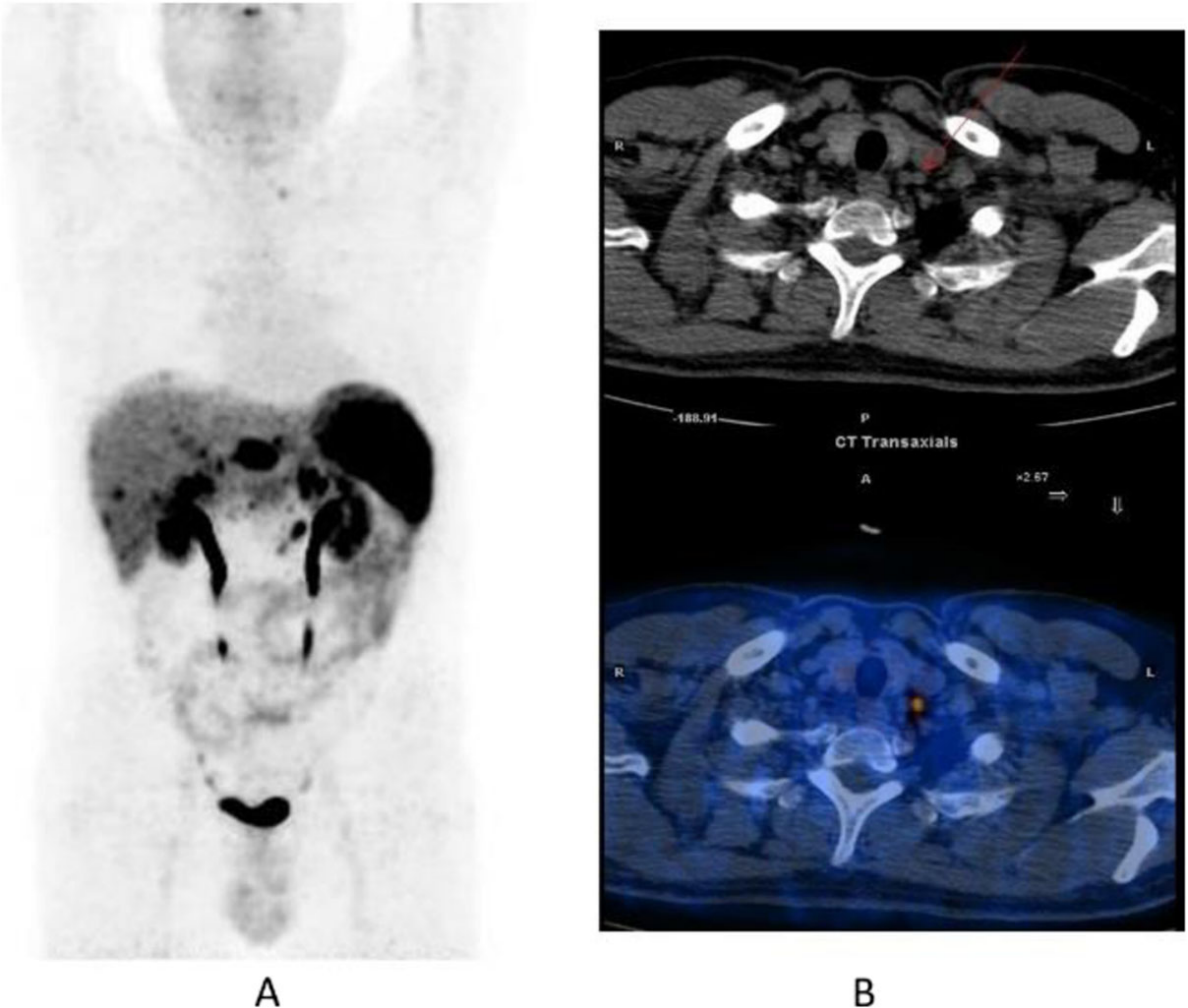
^68^Ga-DOTA peptide, staging NET of the pancreas, sensitivity. *Clinical history*: 60 y.o. man. Incidental finding of a NET of the pancreas grade 2 (Ki67 5%). *PET/CT findings*: multiple areas of increased tracer uptake in the pancreas, liver, and abdominal lymph nodes (a MIP). Note the uptake in a left supraclavicular lymph node 5 mm in size (b CT and fused images)
